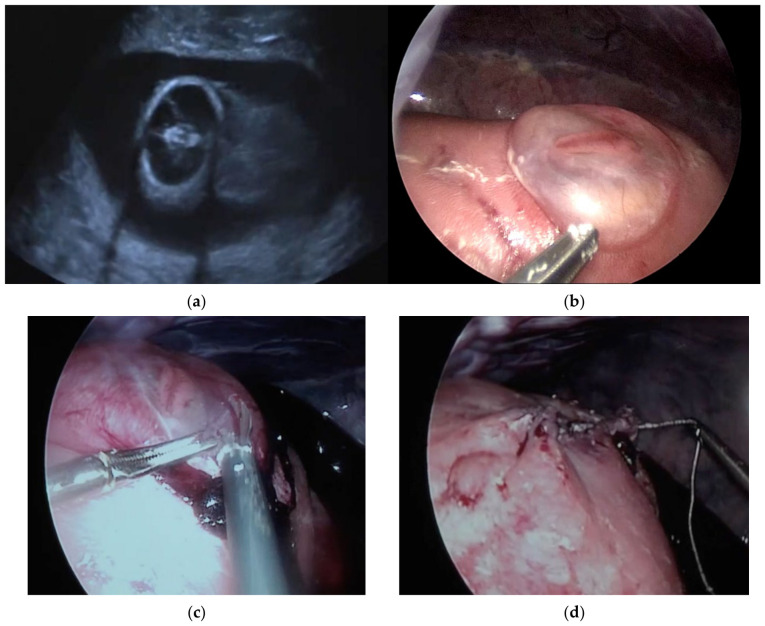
Intrauterine fetoscopic repair of open spina bifida using the SAFER technique: (a) Prenatal ultrasound demonstrating the myelomeningocele sac; (b) Fetoscopic view of the exposed myelomeningocele sac with identification of the neural placode; (c) Dissection and incision of the transitional zone, allowing release and mobilization of the neural placode; (d) Final closure demonstrating a watertight repair.
